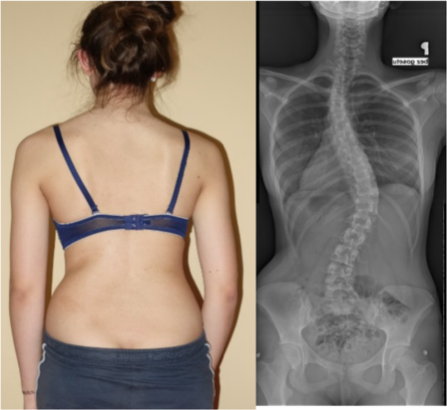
A 15-year-old female patient with a 32° Cobb angle *left upper* thoracic curve and a 36° Cobb angle right thoracolumbar curve as seen on the radiograph on the *right*, has an improved physical appearance and aesthetic with FITS therapy
